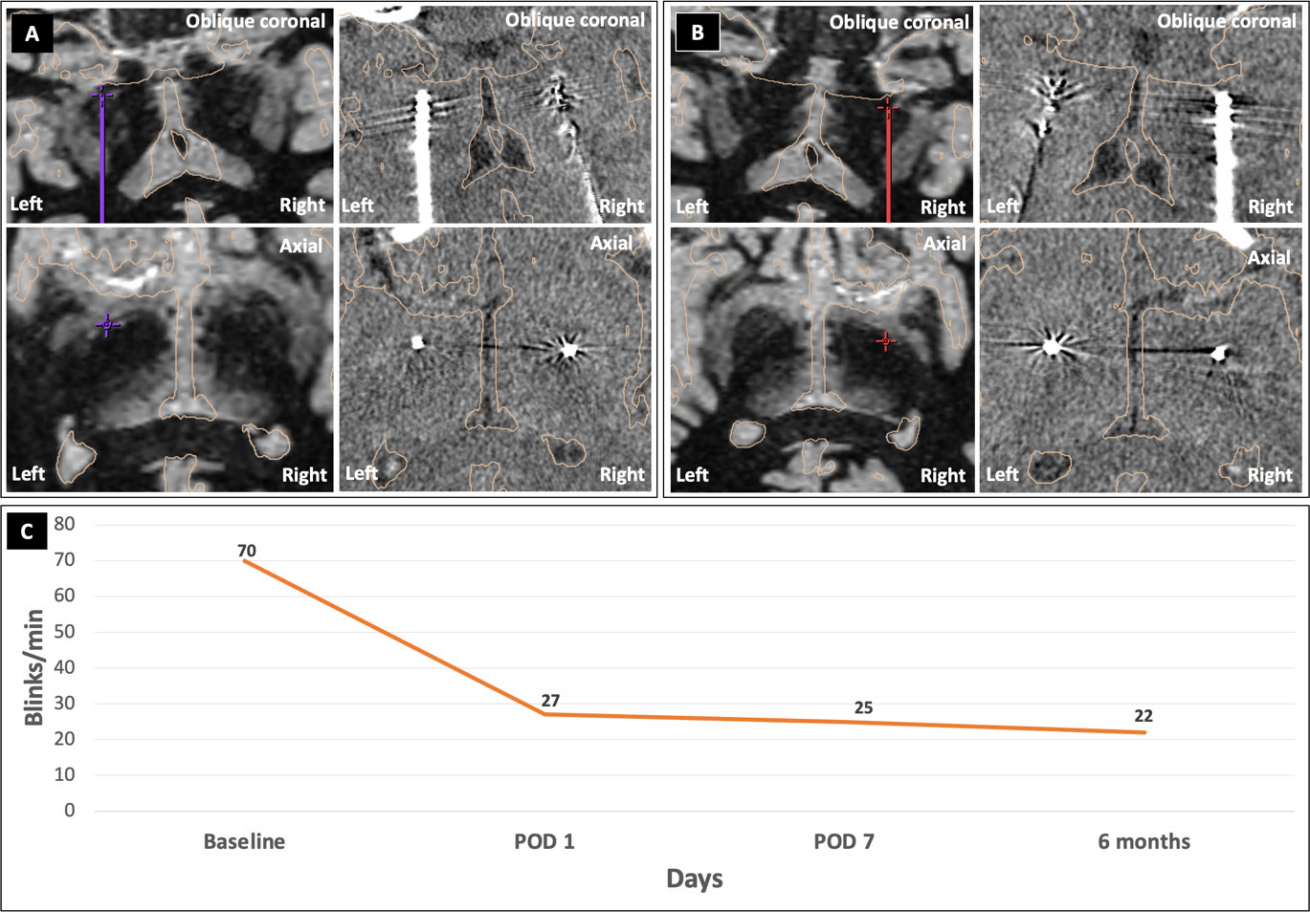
Pre- and postoperative imaging and clinical course. Fast gray matter acquisition T1 inversion recovery magnetic resonance imaging sequences (oblique coronal and axial) to plan lead positioning preoperatively and computed tomography scans (coronal and axial) to evaluate lead placement postoperatively in the (A) left posteroventral globus pallidus interna (pvlGPI) and (B) right pvlGPI. (C) Change in blepharospasm-like tic frequency from before surgery to 6-months after bilateral pvlGP deep brain stimulation.
